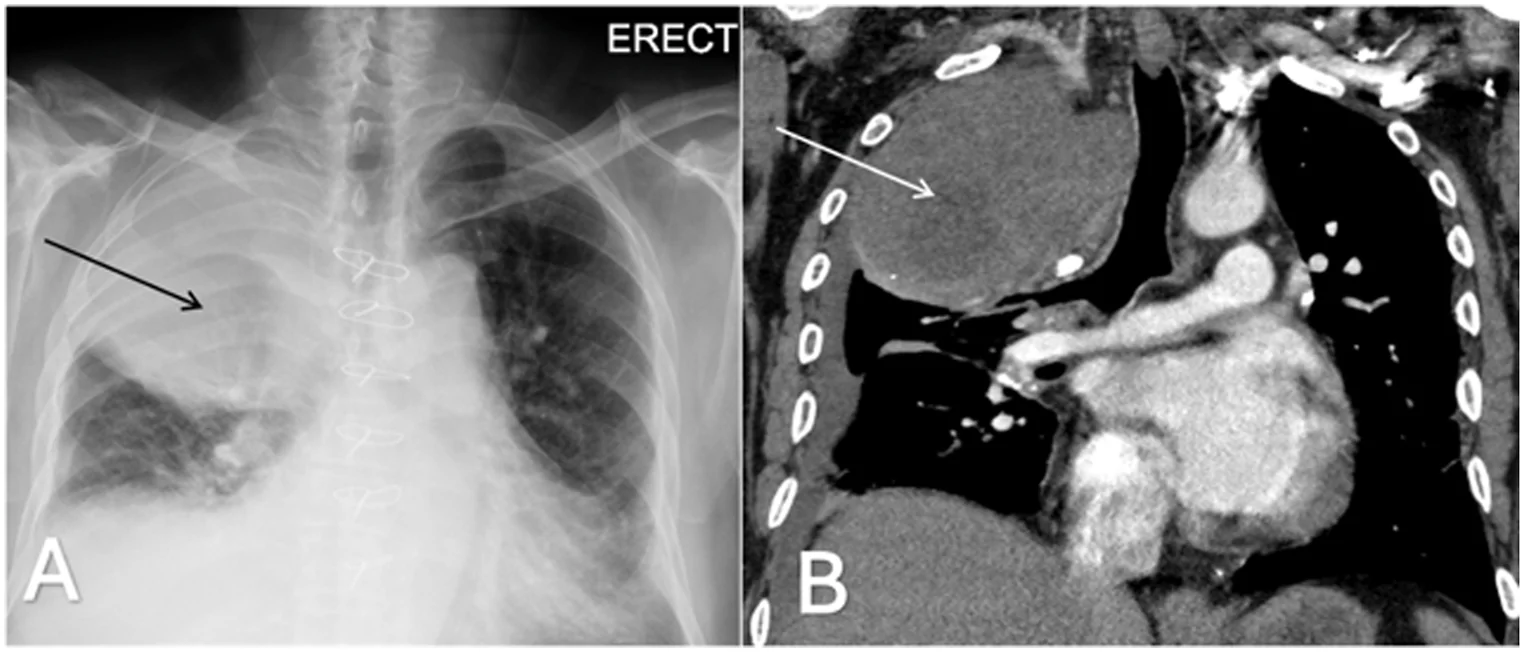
A: PA chest radiograph showing marked interval increase in size of the right upper lobe mass (black arrow). Leftward tracheal deviation indicates mass effect. Post-sternotomy wires are present following pericardiectomy. B: 3 mm coronal soft tissue reconstructed contrast-enhanced CT showing large heterogeneous right upper lobe mass with central areas of low attenuation compatible with tumour necrosis (white arrow) and tracheal deviation.
